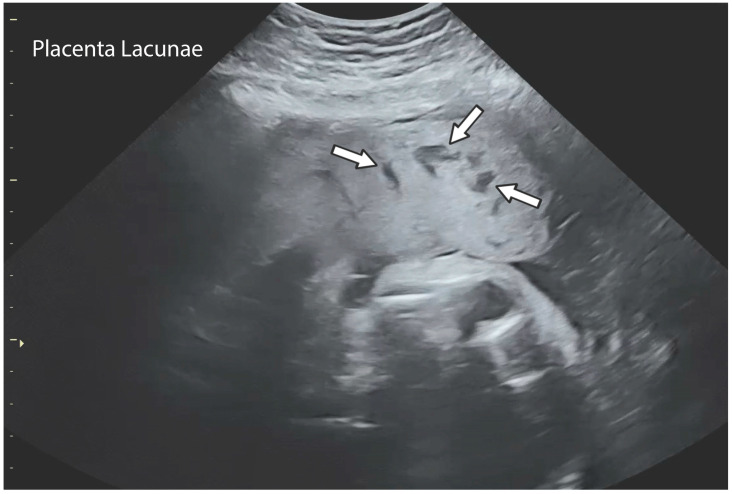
Placental lacunae on ultrasound. The arrows indicate placental lacunae on ultrasound.
